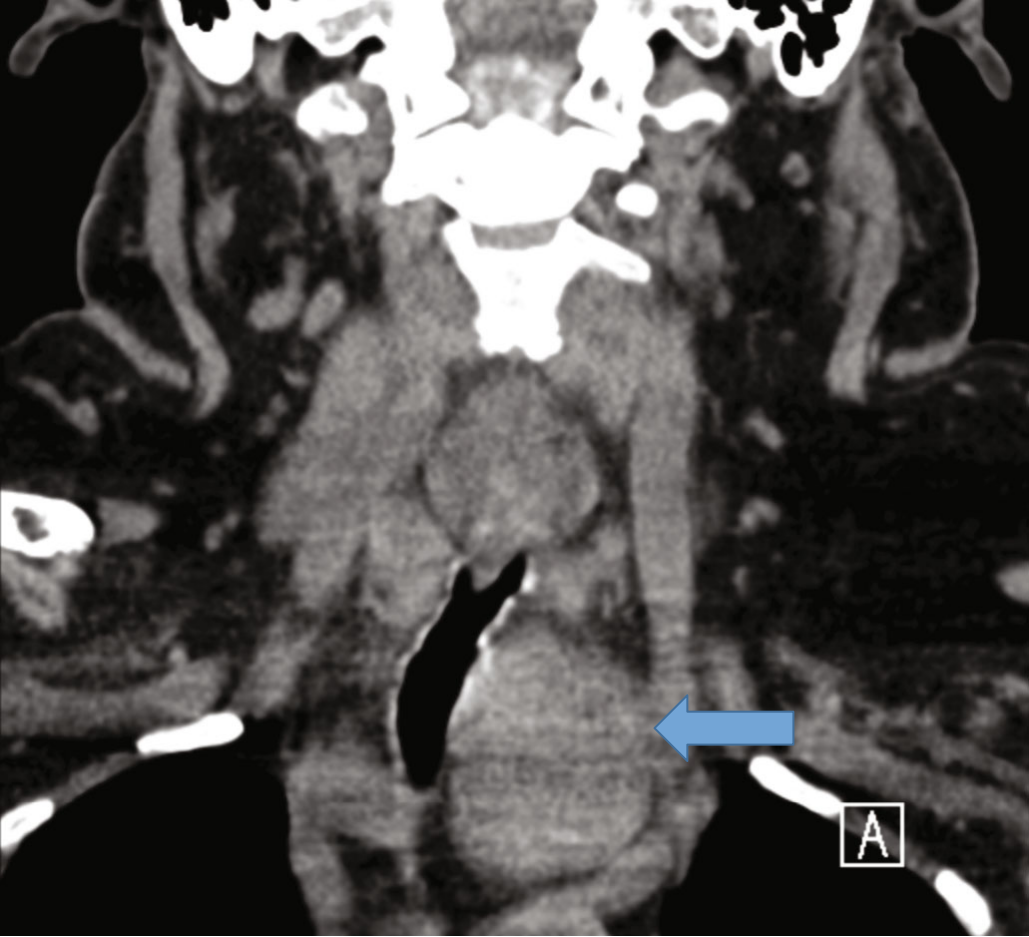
Coronal view of CT neck showing a substernal anterior mediastinal exophytic mass (blue arrow) found to be a benign thyroid mass by US-FNAC measuring 9 × 4 × 3 cm.
